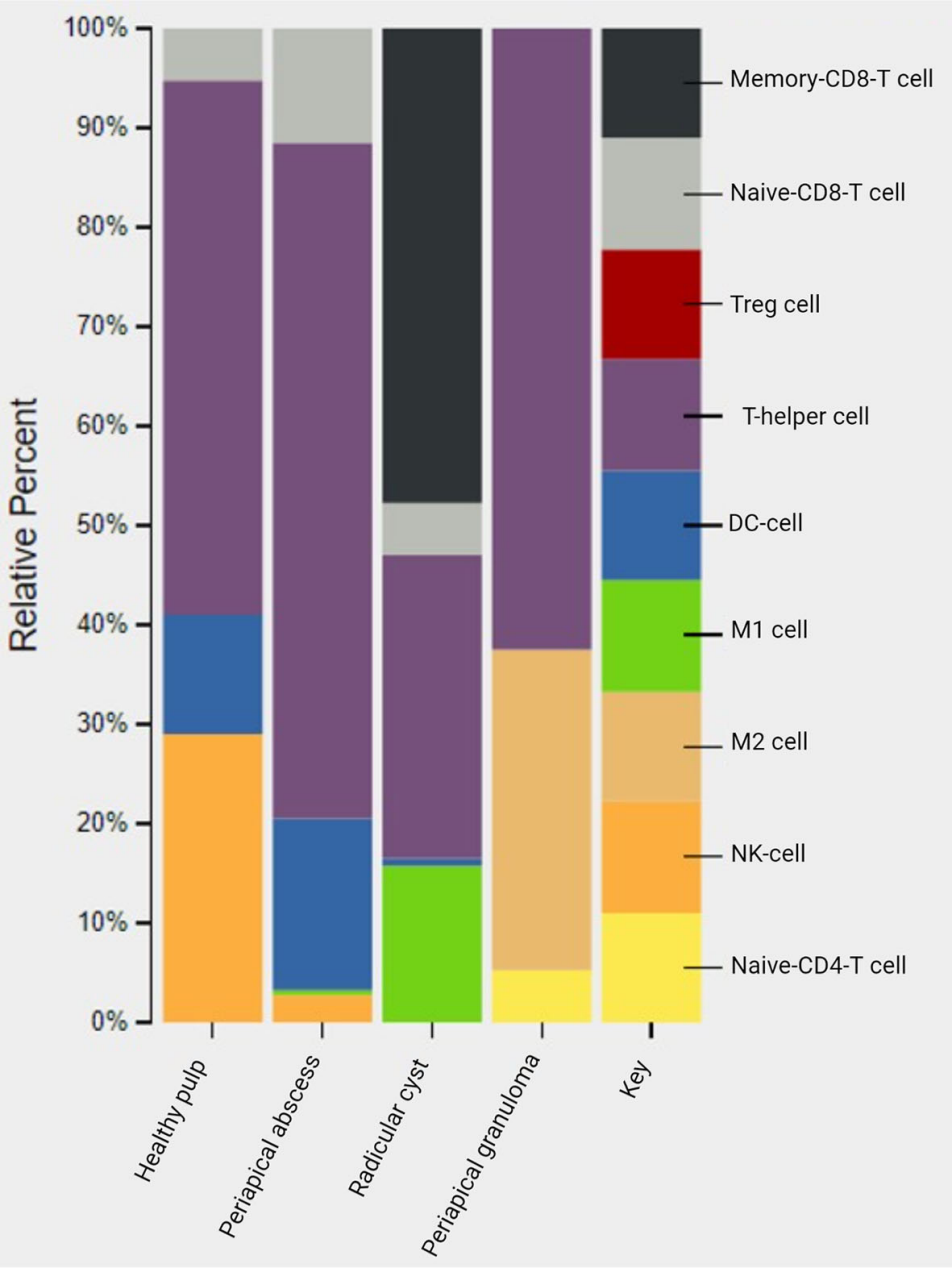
In silico* deconvolution of immune cells of periapical lesions compared with healthy control using CIBERSORT. The color key represented the reference list of immune cell genes. Treg, T-regulatory cell; DC cell, dendritic cell; M1, pro-inflammatory macrophage; M2, anti-inflammatory macrophage; NK, natural killer cell.
